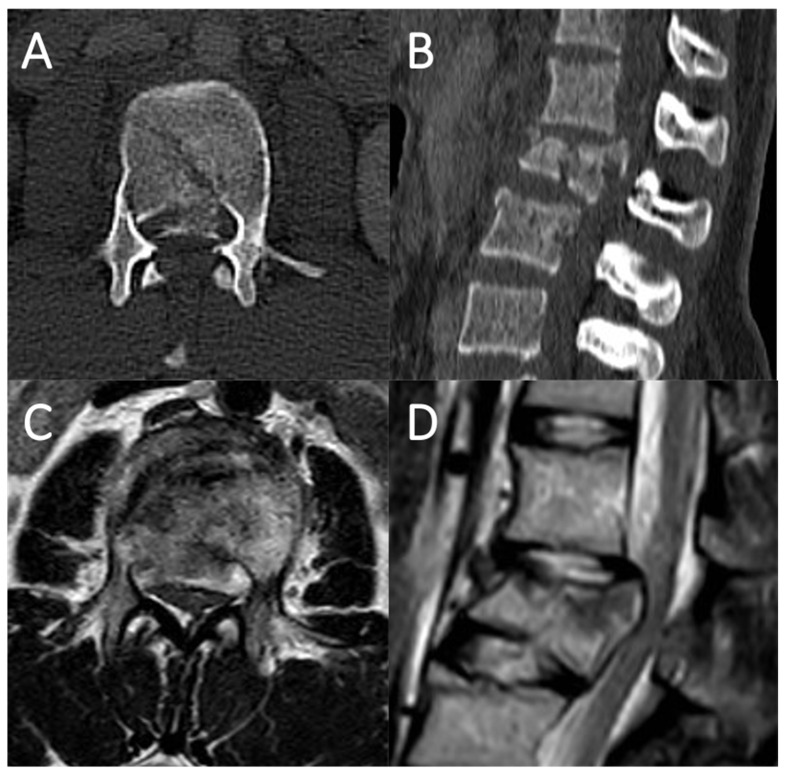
Lumbosacral spine CT-scan ((A), axial view; (B), sagittal view) and MRI ((C), axial view; (D), sagittal view) documenting the L2 type A4 fracture with a single vertebral body fragment, which determined a contusion of conus medullaris, encroaching the spinal canal by over 50%.
